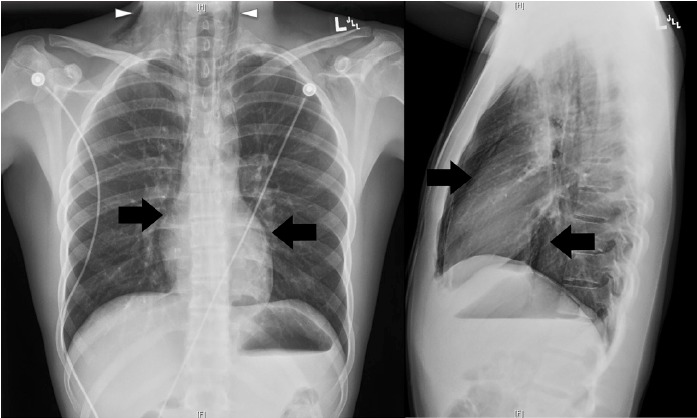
Chest radiograph, PA and lateral views. *PA*, posterior-anterior The patient’s pneumomediastinum was confirmed on chest x-ray, based on the presence of air dissecting along the mediastinum (arrows) and into the lower neck region bilaterally (arrow heads).
